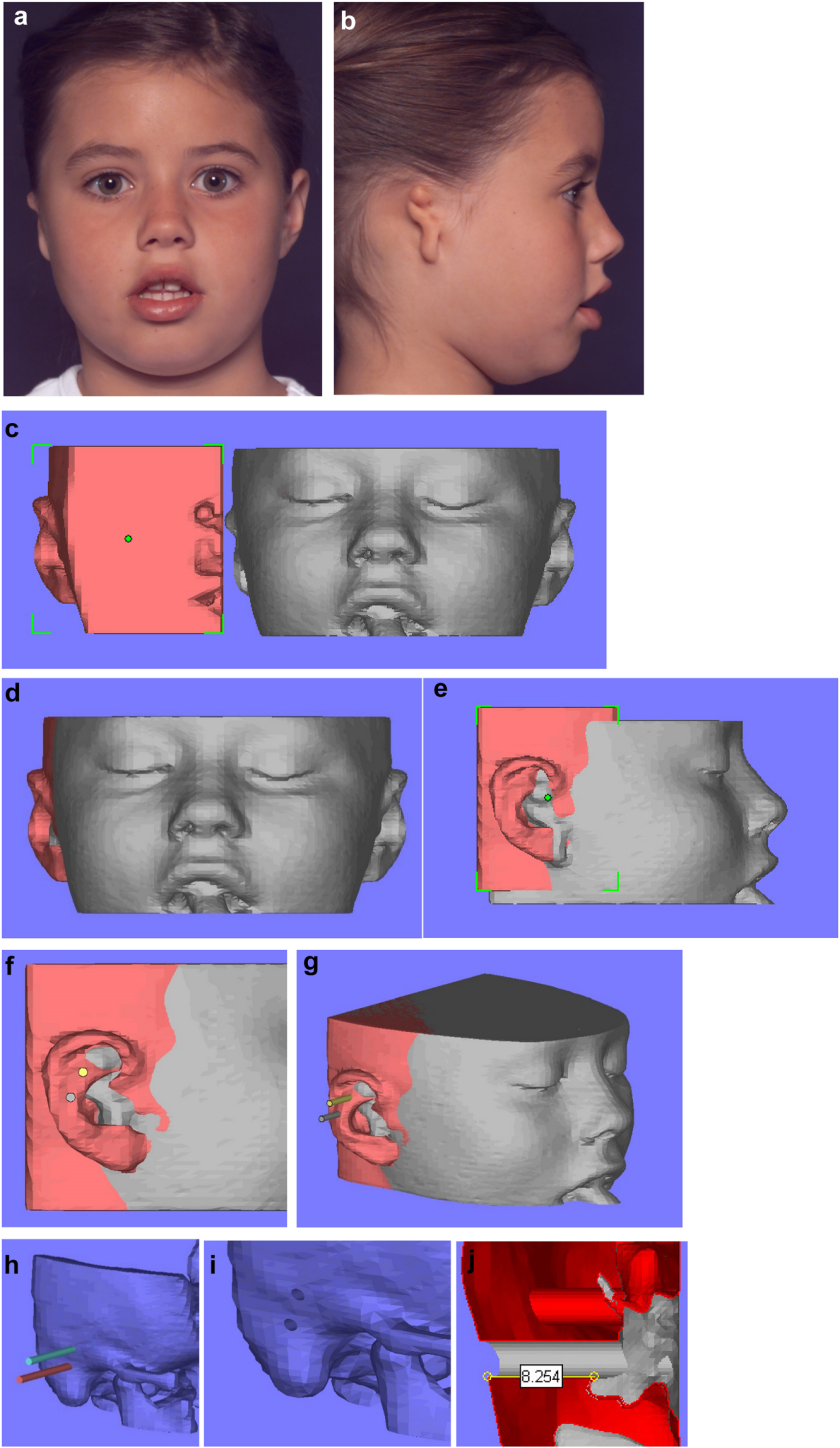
Planning for a child with microtia for a bone anchored ear prosthesis. (a and b) Right microtic ear. (c) CT scan of same patient imported into Mimics. Mirror image created (bone and soft-tissue) of left normal ear and surrounding structures and transferred to microtic side. (d and e) Mirror imaged ear moved until it matches the normal side in all dimensions. (f and g) Implants or cylinders are inserted in the appropriate sites. (h–j) The soft-tissues are then removed from the virtual image, the cylinders removed from the bone, and sections examined of the holes to ensure they have sufficient depth of good quality bone for implant insertion.
